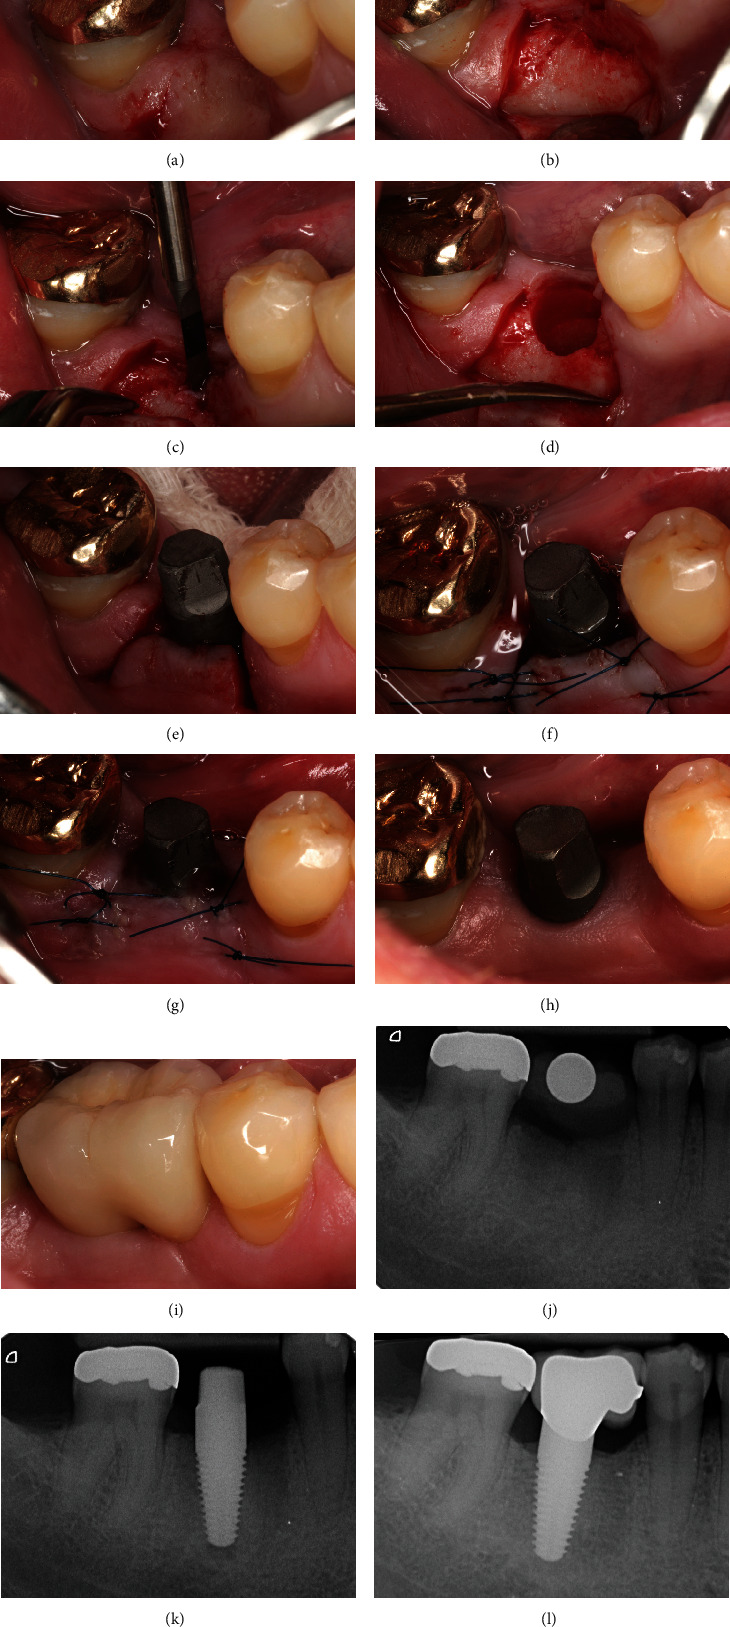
Implantation of one-piece implant (clinical procedure): (a) clinical situation presurgically; (b) elevation of full-thickness flap with preservation of peripheral periodontium at adjacent teeth; (c) implant bed preparation, predrilling instrument; (d) processed implantation site using drilling protocol; (e) placement of one-piece implant with spatial orientation aligned to the natural dentition; (f) apical repositioned and sutured flap; (g) clinical situation one week after surgery; (h) clinical situation 12 weeks after surgery; (i) single crown prosthetic restoration with mesial pontic design; (j) presurgical radiographic control, calibration ball for planning of implantation; (k) postsurgical radiograph taken directly after implant placement; (l) radiographic control nine years after treatment.
